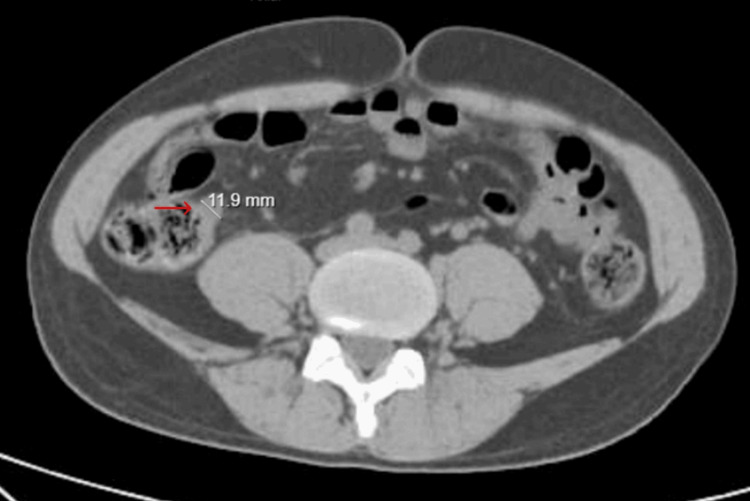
Abdominopelvic computed tomography (CT) findings Axial contrast-enhanced abdominal CT scan showing an enlarged appendix measuring approximately 11.9 mm in diameter with peri-appendiceal fat stranding (arrow), consistent with acute appendicitis diagnosis.
